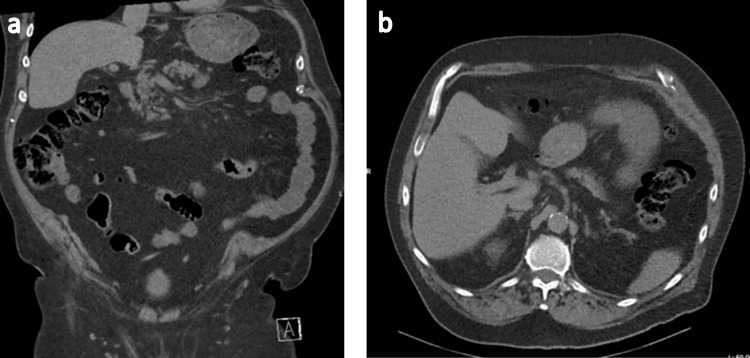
Control CT scan eight months after surgery. No recurrence was observed in frontal view (a) nor in sagittal view (b).
